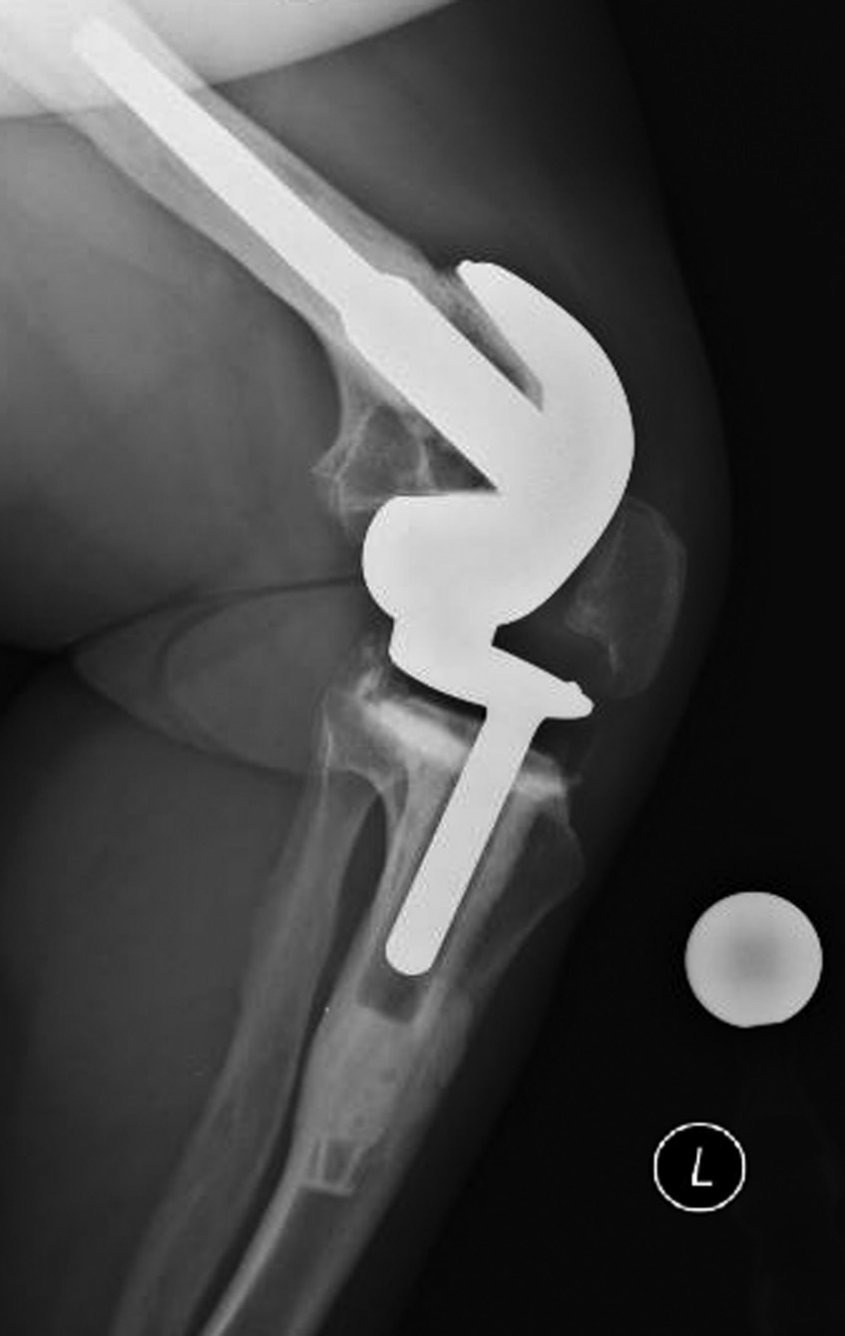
AP bone length, right and left lateral, and sunrise radiographs 2 years postoperatively with no evidence of early polyethylene wear, aseptic loosening, or stem subsidence.
